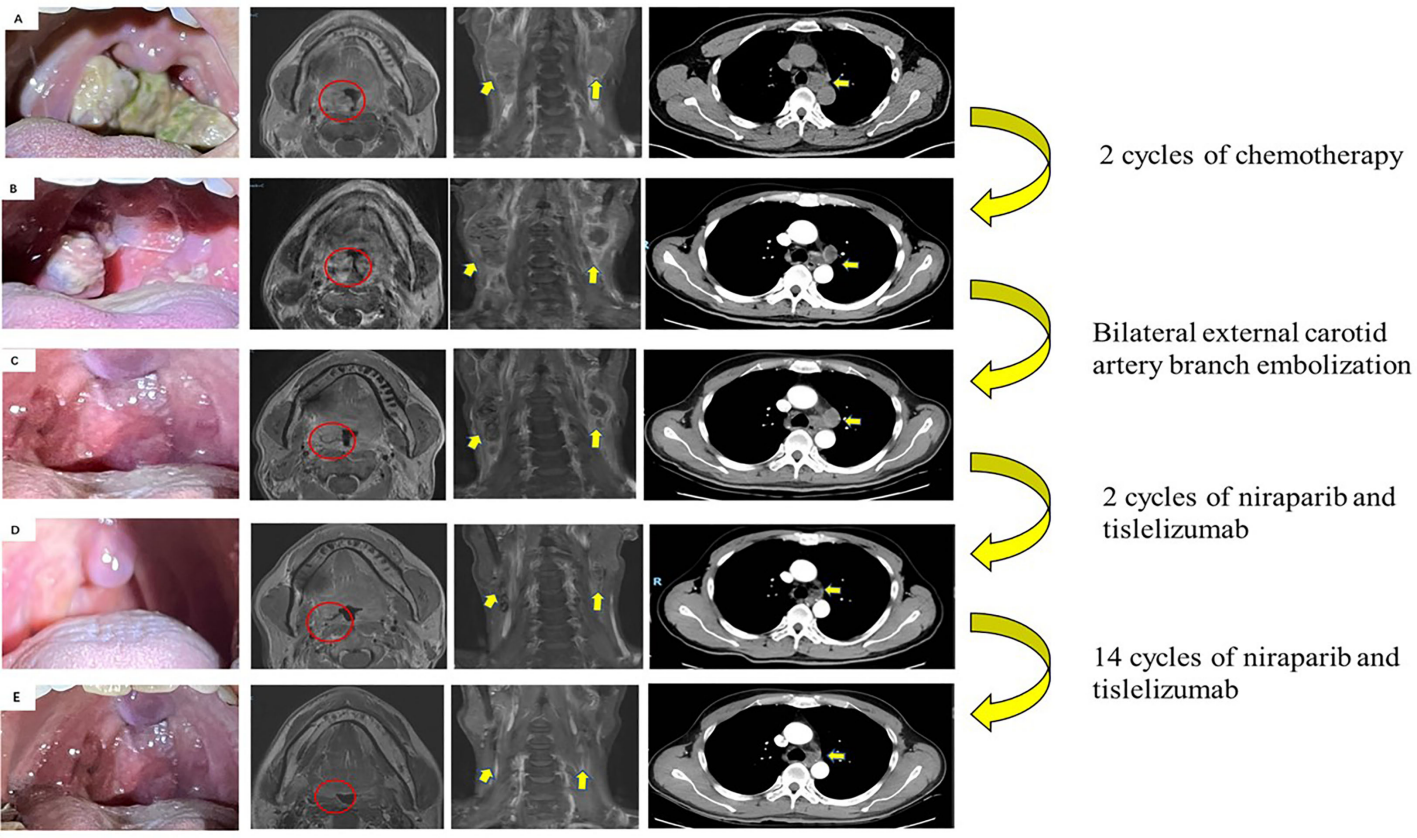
Images of primary tumor and metastatic lymph nodes during therapy. (A) Initial scans showed a mass in the right tonsil (red circle). Enlarged lymph nodes in the neck and mediastinum (yellow arrows) (December 2020). (B) Disease progression after 2 cycles of chemotherapy by RECIST criterion (February 2021). (C) After bilateral external carotid artery branch embolization (March 2021). (D) Partial response after 2 cycles of niraparib and tislelizumab by RECIST criterion (May 2021). (E) Re-examination of MRI and CT revealed no evidence of progression to the tonsils, cervical lymph nodes, and mediastinal and hilar lymph nodes (May 31, 2022).
